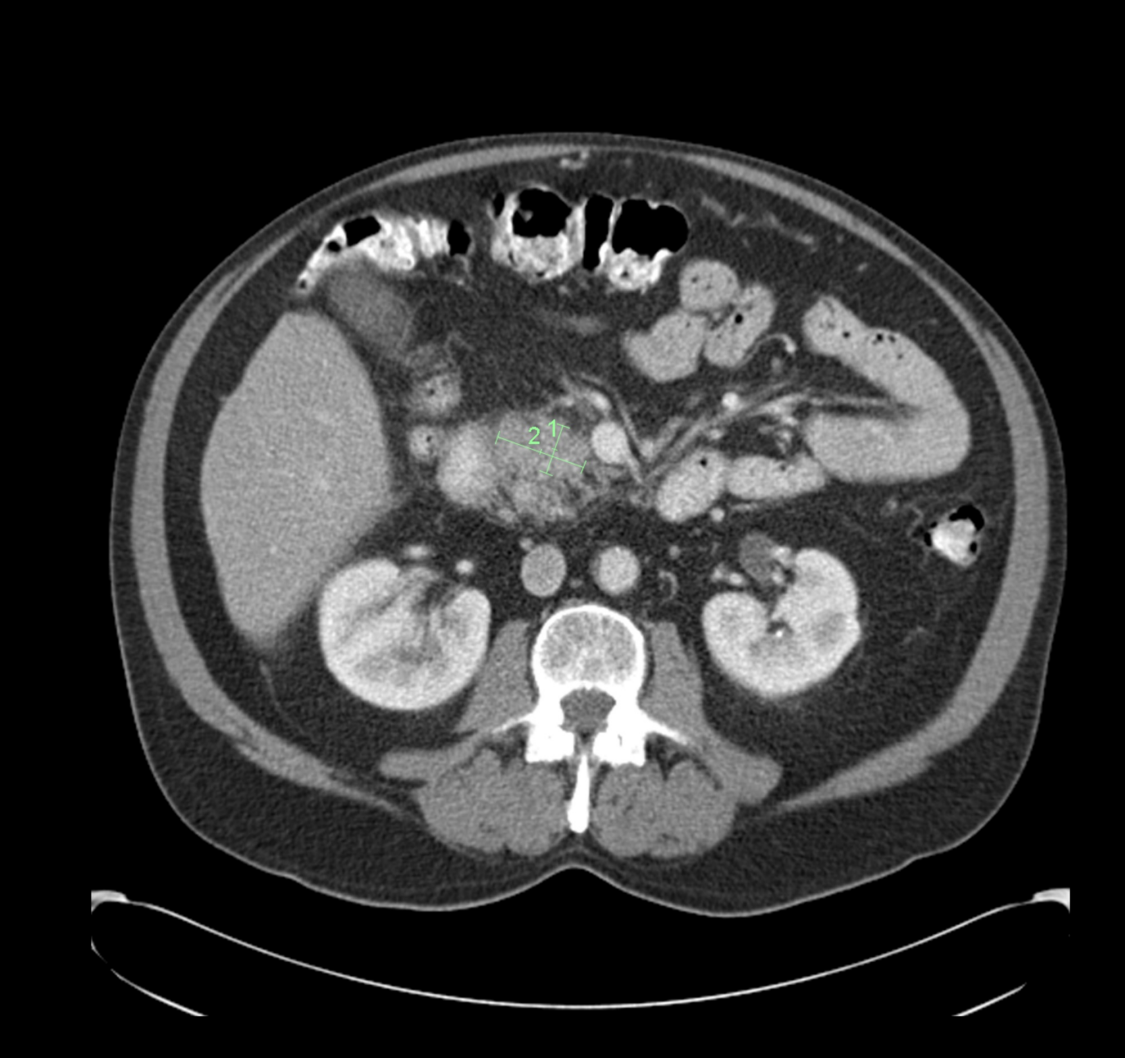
CT abdomen pelvis showing 2.0 x 1.0-cm ill-defined solid mass in the head of the pancreas CT, computed tomography
